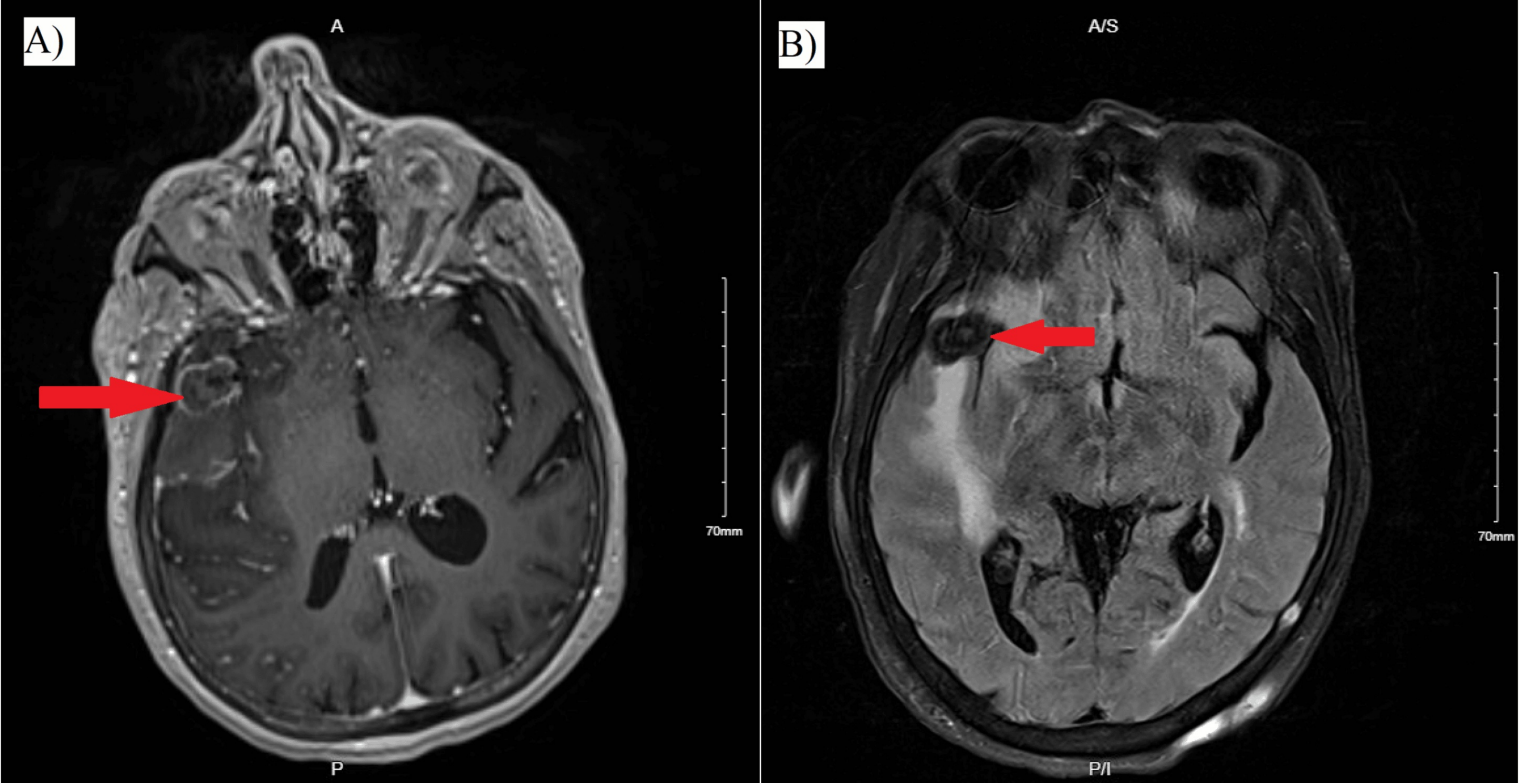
(A) Axial T1-weighted MRI showing a hypointense, peripherally enhancing large thrombosed right MCA bifurcation aneurysm, causing approximately 3 mm of leftward midline shift. (B) GRE sequence MRI demonstrating high signal intensity corresponding to the thrombosed aneurysm. GRE: gradient recalled echo; MCA: middle cerebral artery
